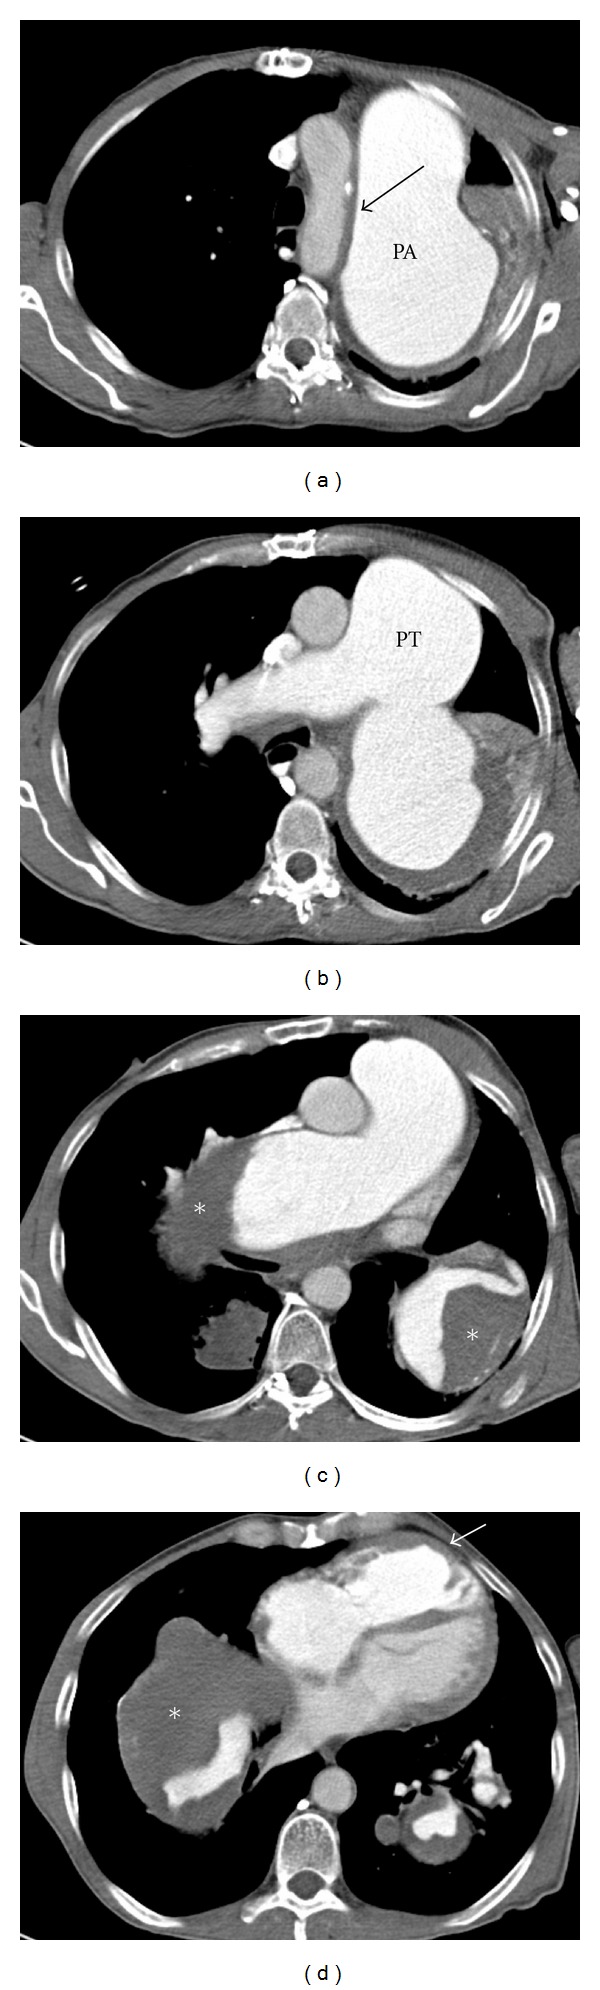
Chest CT featured the signs of pulmonary arterial hypertension, pulmonary trunk (PT) dilatation, aneurysmal dilatation of the central pulmonary arteries (PA), wall-adherent thrombotic material in pulmonary arteries (asterix), right ventricular hypertrophy (white arrow in (d)). No mass lesion or lymphadenopathy was found and the radiologist suggested the cardiovocal syndrome as a possible diagnosis because of the obvious mass effect caused by the dilated central pulmonary arteries. The possible compression of the left recurrent laryngeal nerve between the aorta and pulmonary artery is well appreciated (black arrow in (a)).
